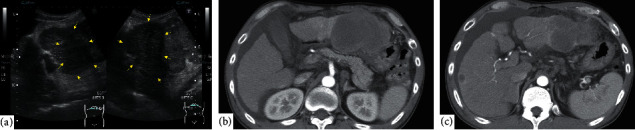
(a) Abdominal ultrasound showing a hypoechoic, mosaic-like, giant mass in the lateral segment of the left hepatic lobe of the cirrhotic liver (arrows). (b), (c) Early arterial phase of a contrast-enhanced computed tomography scan showing an 8 cm hypodense mass in the left hepatic lobe, a hypodense mass with ring-like enhancement in the right lobe, and multiple swollen lymph nodes.
